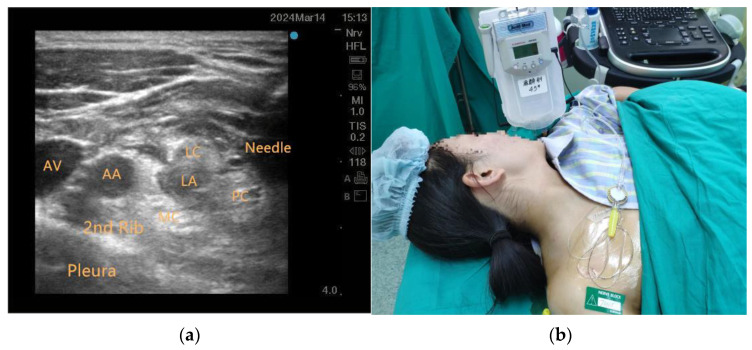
Ultrasound-guided BPB and postoperative analgesia in CBPB group. (a) Ultrasound image of the costoclavicular BPB; (b) PCA-connected catheter secured to thoracic skin; AA = axillary artery; AV = axillary vein; LC = lateral cord; MC = medial cord; PC = posterior cord; LA = local anesthetic.
